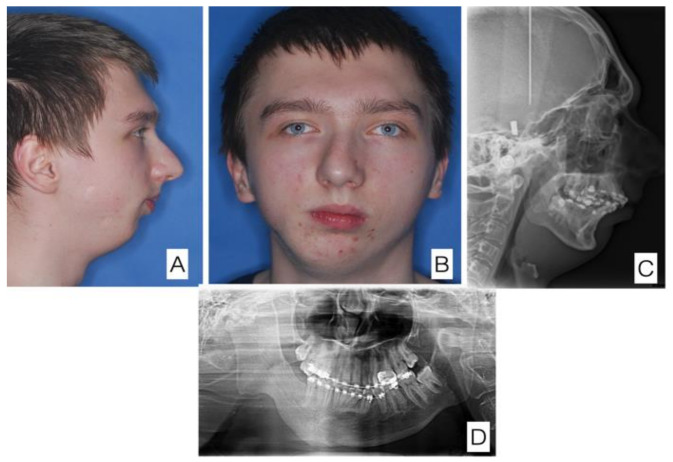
A 15-year-old patient with temporomandibular joint ankylosis on the right side after *Staphylococcus aureus* infection at age 5. Condition prior to osseodistraction of the right mandibular ramus (the first stage of the treatment). (A) Lateral view of the face, (B) anterior view of the face, (C) cephalometry, (D) orthopantomogram.
